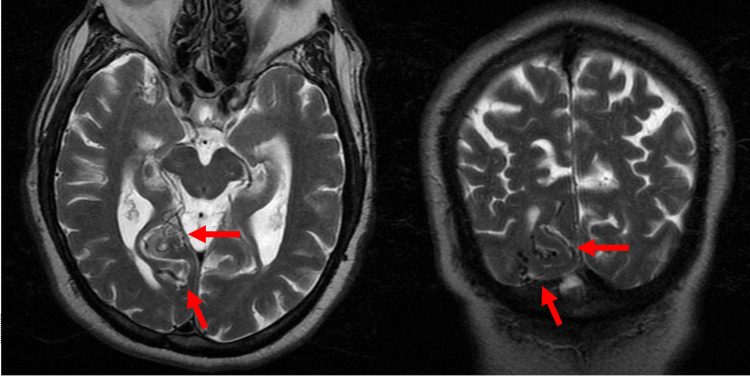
T2-weighted MRI The (a) axial and (b) coronal planes demonstrate subtle subarachnoid serpiginous vascular flow voids (arrows)
